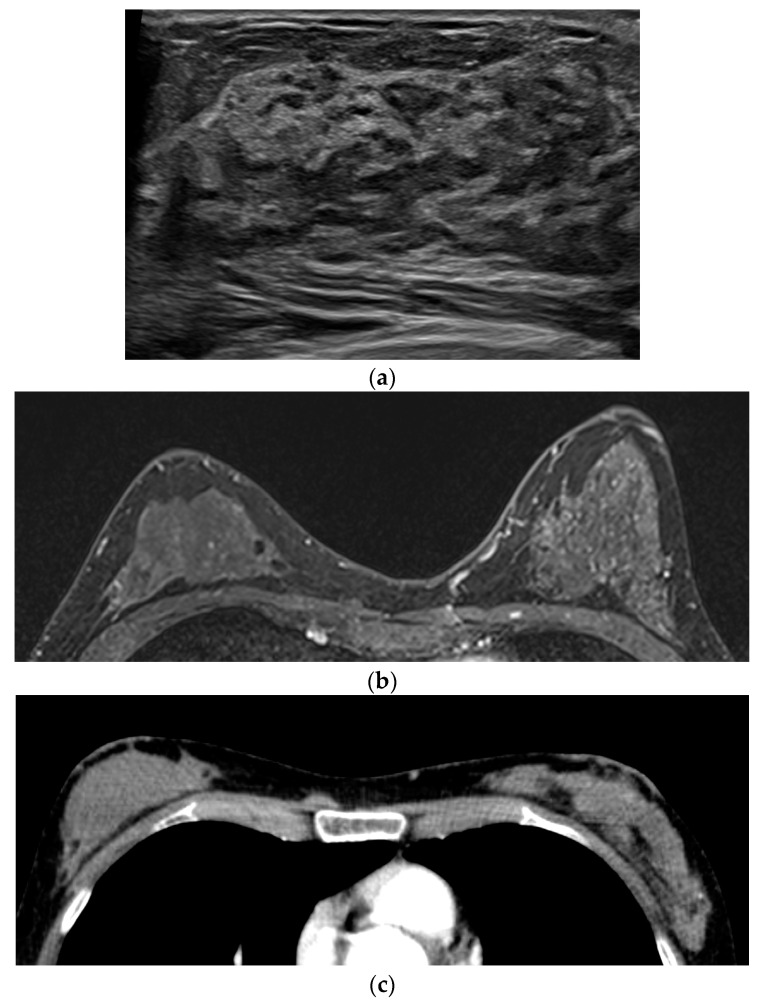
A 42-year-old woman with screening. (a) In the ultrasound, there was a 5.5 cm extent diffuse nonmass lesion at the right upper breast, and this lesion was confirmed as an invasive lobular carcinoma on core needle biopsy. (b) No discernible enhancing lesion at the right upper breast in the early phase of dynamic contrast-enhanced T1WI. (c) Also, no detectable enhancement at the correlating site in the contrast-enhanced chest CT.
